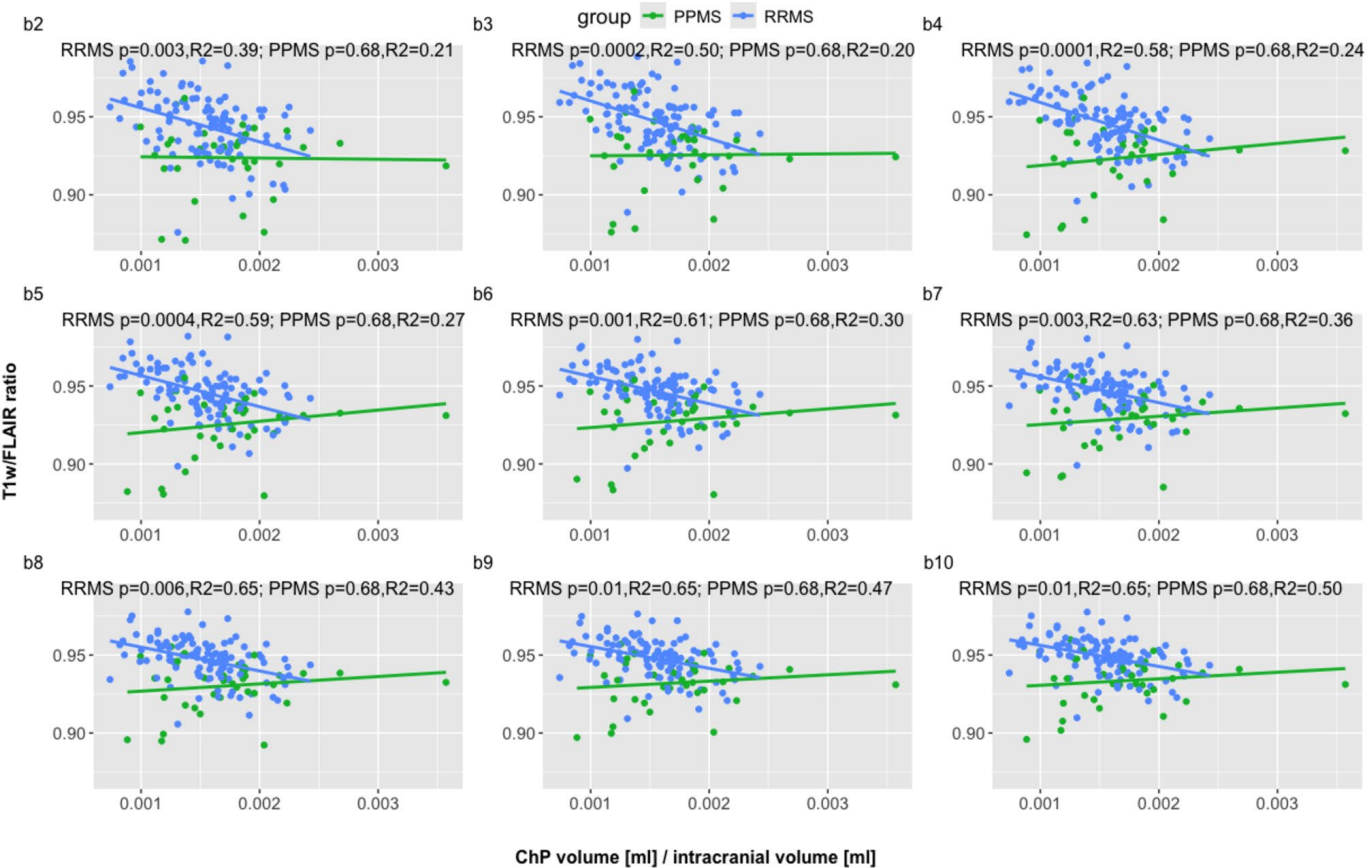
T1w/FLAIR ratios versus ChP volumes for each periventricular band. Relationship between T1w/FLAIR ratios and ChP volumes normalized to intracranial volume in each periventricular band (band b2 to band b10) for relapsing-remitting MS (RRMS,blue) and primary progressive MS (PPMS, green) patients. *P*-values (for ratios vs. ChP volumes) and adjusted R2 were obtained from linear regression analyses with age, sex, and center as covariates. P-values were corrected for multiple comparisons using Benjamini-Hochberg procedure
